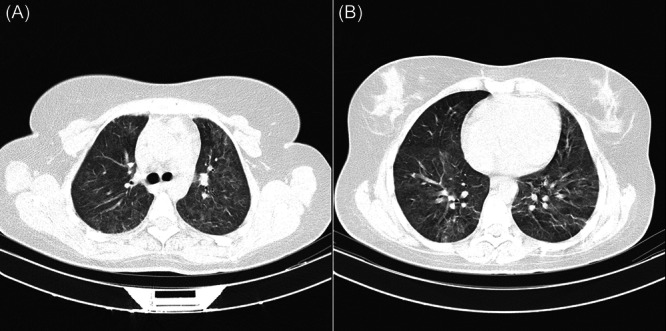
Chest CT Scan of an immunocompromised patient with diffuse involvement of the lung. These pictures, show 34‐year‐old immunocompromised female patient with diffuse involvement of all five lobes of her lung, this pattern was significantly higher among immunocompromised patients. All 5 lobes of this patient's lung (Shown in A and B) scored 5/5 based on the semiquantitative scoring system with a sum of 25/25 showing the severity of lung involvement in this patient.
